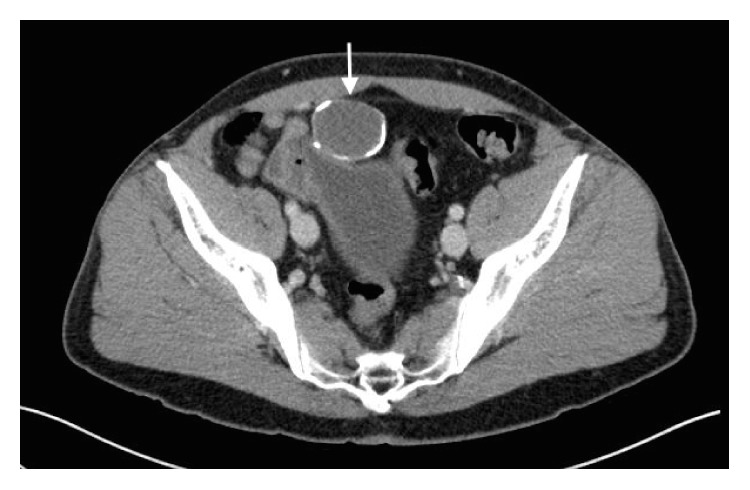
Computed tomography scan showing a cystic lesion anterior to the bladder with calcification within the wall (arrow).
